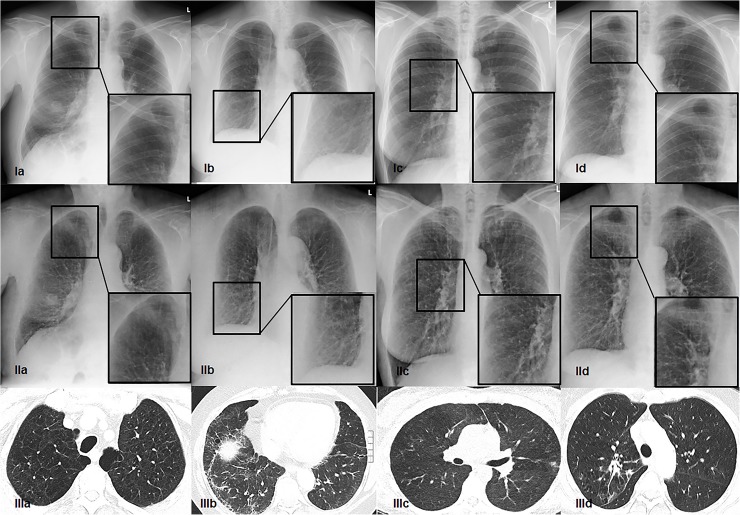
Interstitial lung changes in different imaging modalities. Appearance of interstitial lung changes such as (a) emphysema, (b) reticular lung changes, (c) nodular lung changes and (d) scarring of lung parenchyma with (I) CR, (II) DESR soft tissue image and (III) CT in four different patients. While nodular changes are visible in all the three imaging modalities (Ic, IIc and IIIc), emphysema, reticular lung changes and lung scarring are better depicted in the DESR soft tissue image (IIa, IIb and IId) compared to the CR image (Ia, Ib and Id). The patient having the emphysema had also a solid lung mass in the right lower lobe (Ia and IIa).
